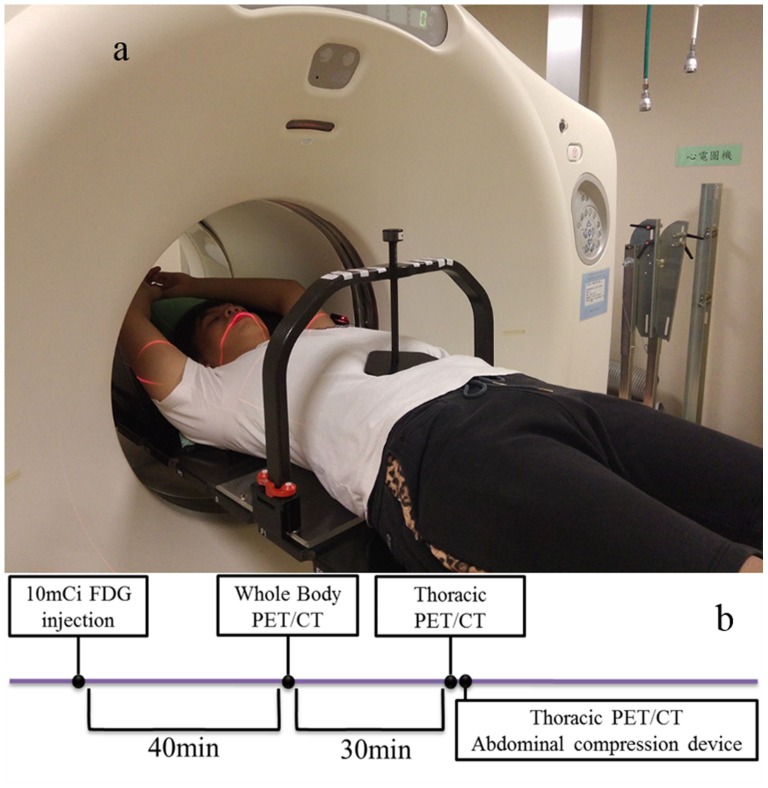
Patient setup with the abdominal compression device (a) located in the upper abdomen region to limit the amount of respiration. (b) The data acquisition protocol for the PET/CT imaging of patients with abdominal compression immediately after conducting free-breathing imaging.
